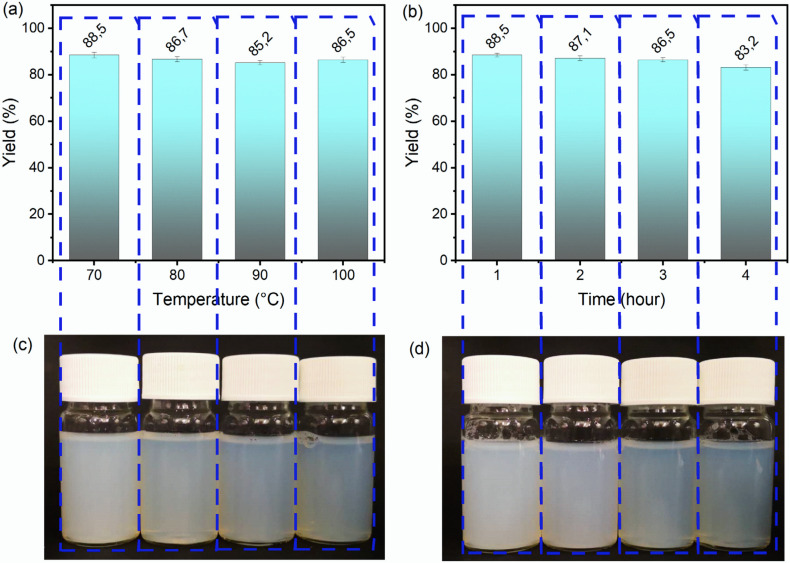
Mass yield of ChNCs after DES treatment (and ultrasonication) at different temperatures (3-h treatment) (a) and for different reaction time (at 100 °C) (b) and the appearance of the corresponding suspensions (c,d).
